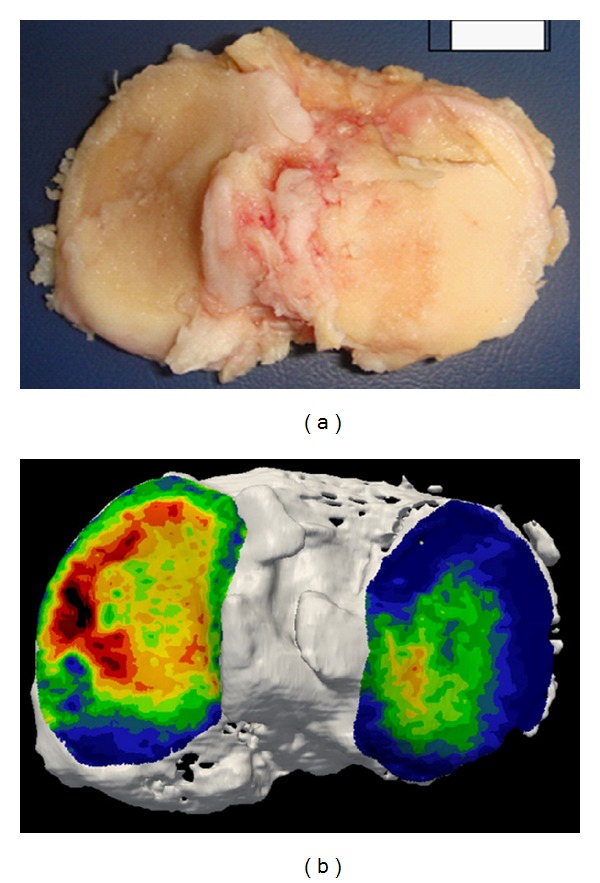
Tibial plateau of an elderly patient showing end-stage OA with complete cartilage loss (a). Computed tomography osteoabsorptiometry (CT-OAM) indicates high bone density in red (b).
